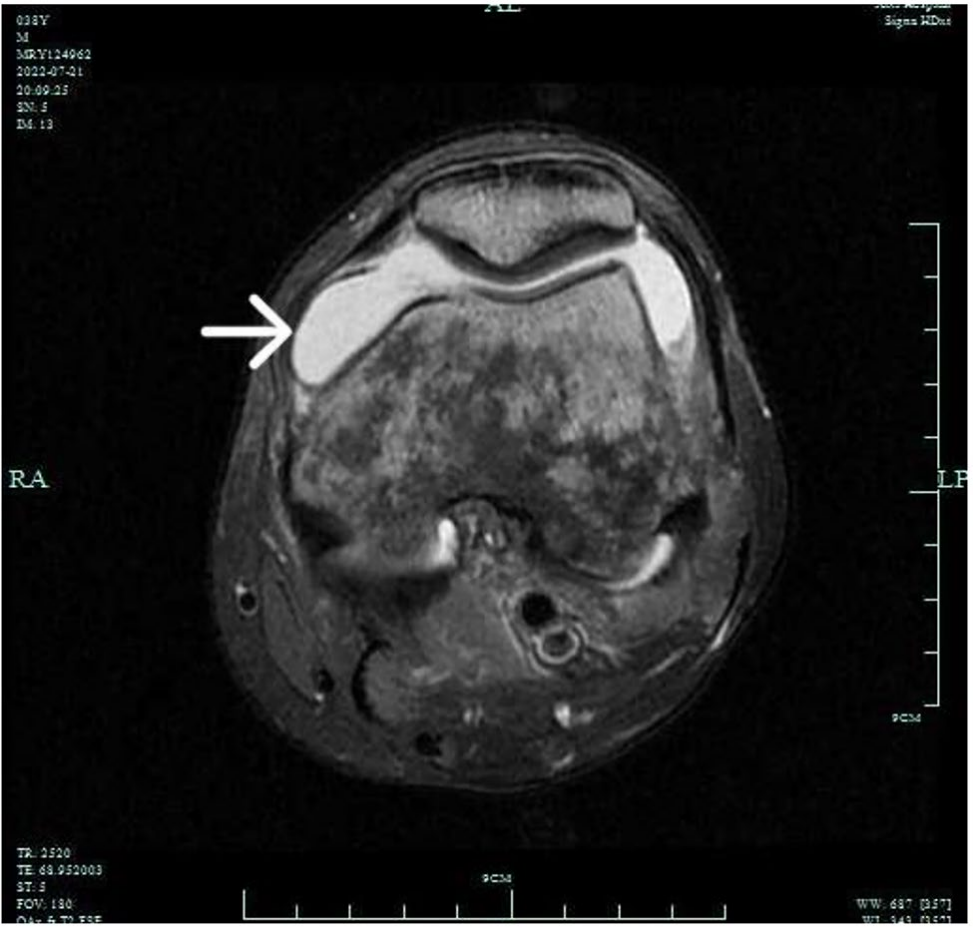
Transverse axis MRI of the left knee joint revealed numerous patchy long T1 and long T2 signal lesions in the knee bones, with indistinct edges. Abundant fluid signals were noted in the joint space and upper abdominal bursae, accompanied by swelling of the surrounding muscles and soft tissues
